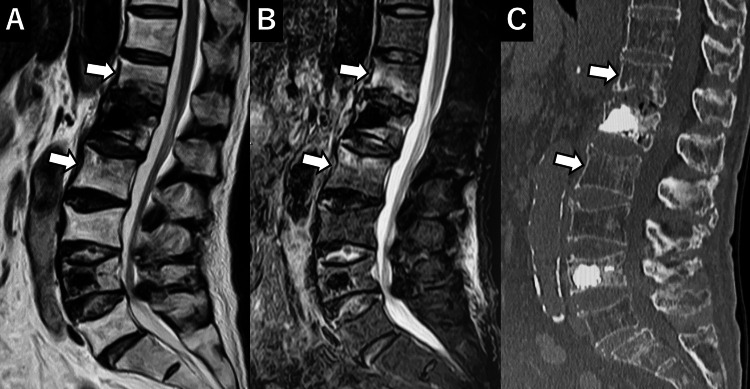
Pre-second surgery MRI and CT A: T2-weighted image (T2WI), B: Short T1 inversion recovery (STIR), C: CT. New vertebral compression fractures are noted at Th12 and L2 (arrows).
